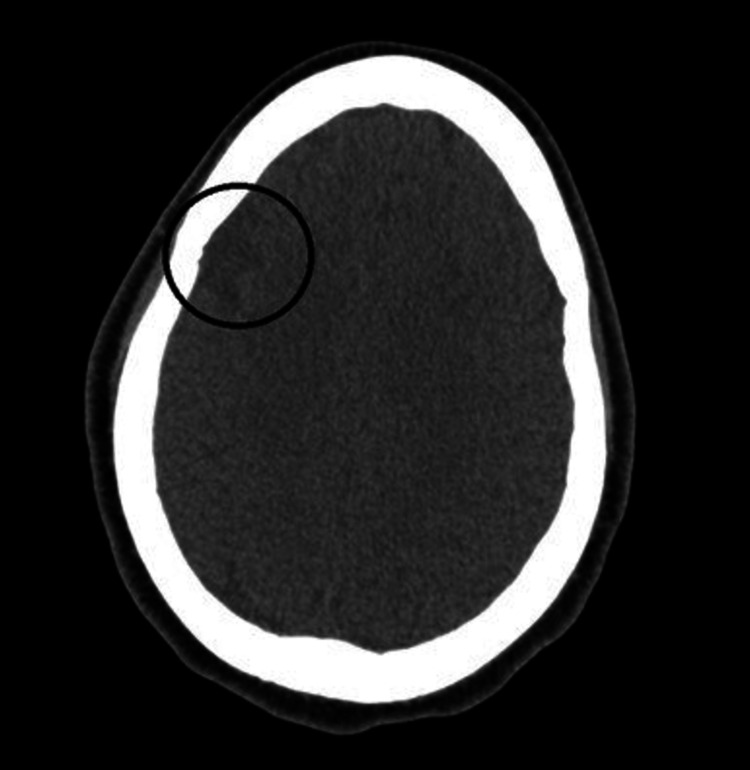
Computed tomography head showing lateral right frontal lobe encephalomalacia (circled)
